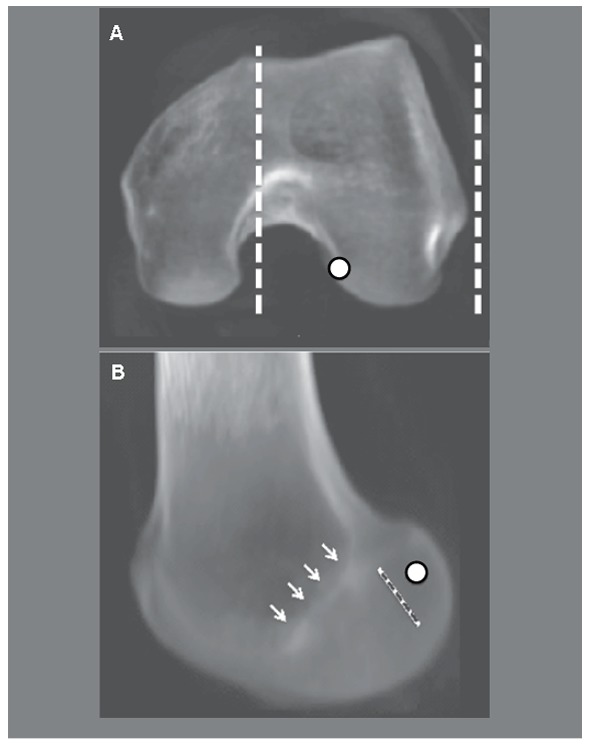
Transparent CT scan of the lateral femoral condyle. A) Dotted line: limit of the lateral femoral condyle including the intercondylar notch. B) Sagittal view of the selected lateral condyle. White arrows: Blumensaat line; dotted line: resident ridge; circle: central ACL footprint.
